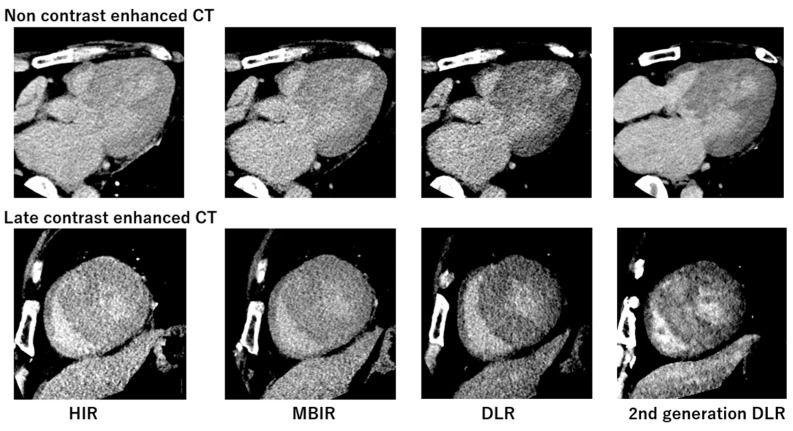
Comparison of non-contrast and late contrast-enhanced CT images using four reconstruction methods in a patient with hypertrophic cardiomyopathy.
